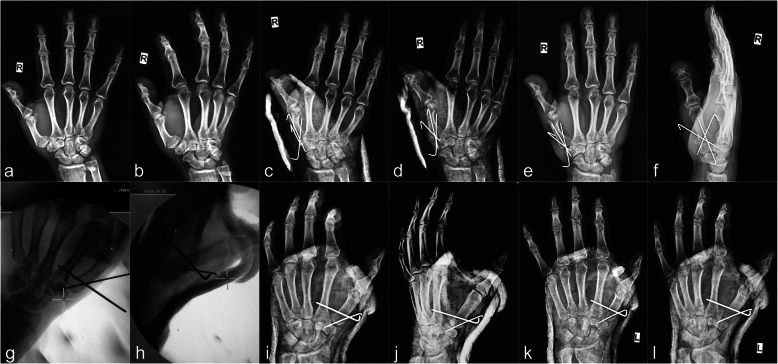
Representative cases of the traditional technique of percutaneous K-wire fixation. a–f Preoperative and postoperative follow-up radiographs of Patient 22. g–l Intraoperative fluoroscopy and postoperative follow-up radiographs of Patient 23
